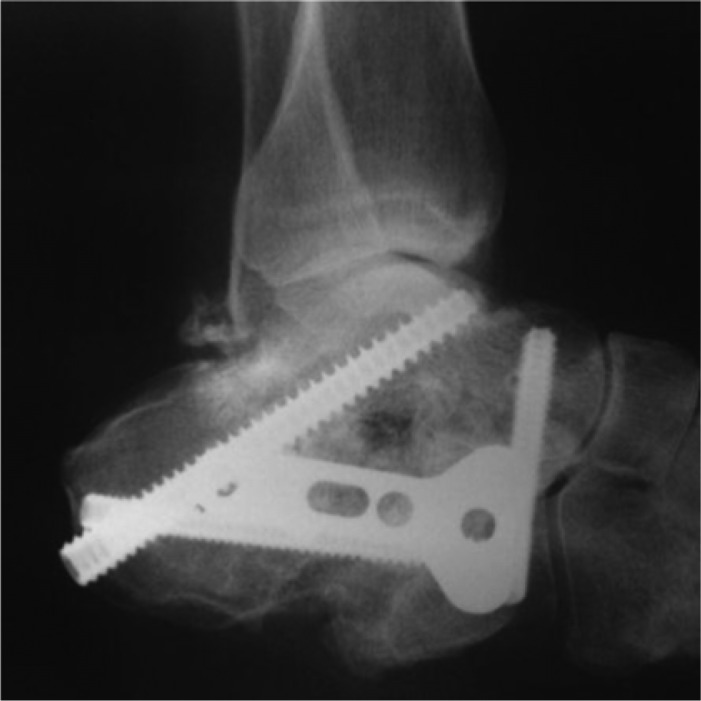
Plain radiographic lateral ankle view showing a subtalar joint arthrodesis with rigid internal fixation consisted of plate and screws.
